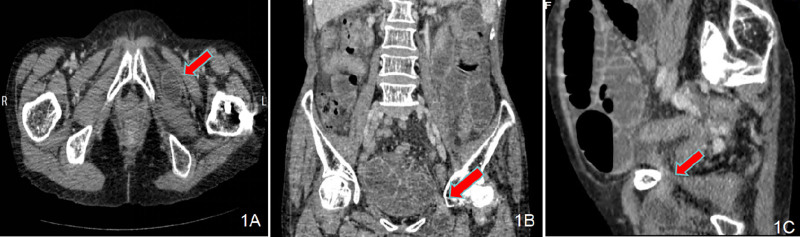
(A) Contrast-enhanced CT imaging showing the hernia sac (red arrow) between the obturator externus and the pectineus muscles passing through the left-sided obturator foramen. (B and C) A herniation of the small bowel into the left obturator canal (red arrow), with features of small intestine obstruction in the coronal and sagittal views.
